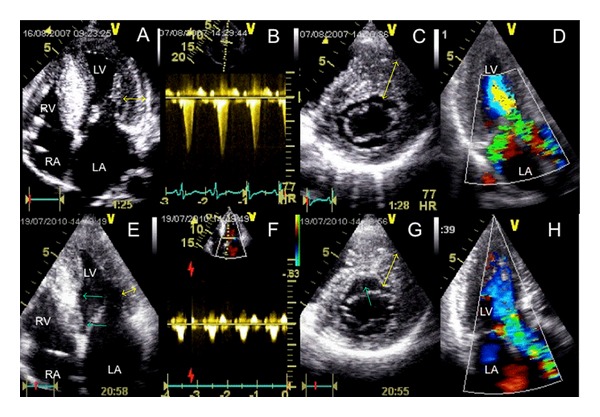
Upper panel (A–D): HOCM with severe hypertrophy of the left ventricle at baseline, particularly involving the septum ((A) and (C)) and exceeding the “critical” threshold of 30 mm (double arrow in (C)). (A) four-chamber view, (B) cw-Doppler profile of LVOT flow with a resting gradient of 70 mm Hg, (C) short-axis view in diastole with double arrow pointing to anterior septal hypertrophy, (D) apical long axis with the turbulent LVOT jet and the obstruction-associated mitral regurgitation. Lower panel (E–H): two years after successful septal ablation which has left a myectomy-like trough in the basal septum (arrows in (E) and (G)) and completely eliminated outflow gradient (F) and mitral regurgitation (H), regression of left ventricular hypertrophy can be observed both in the septum (double arrows in (G)), and the lateral wall (double arrow in (E)). LA: left atrium RA: right atrium RV: right ventricle LV left ventricle.
